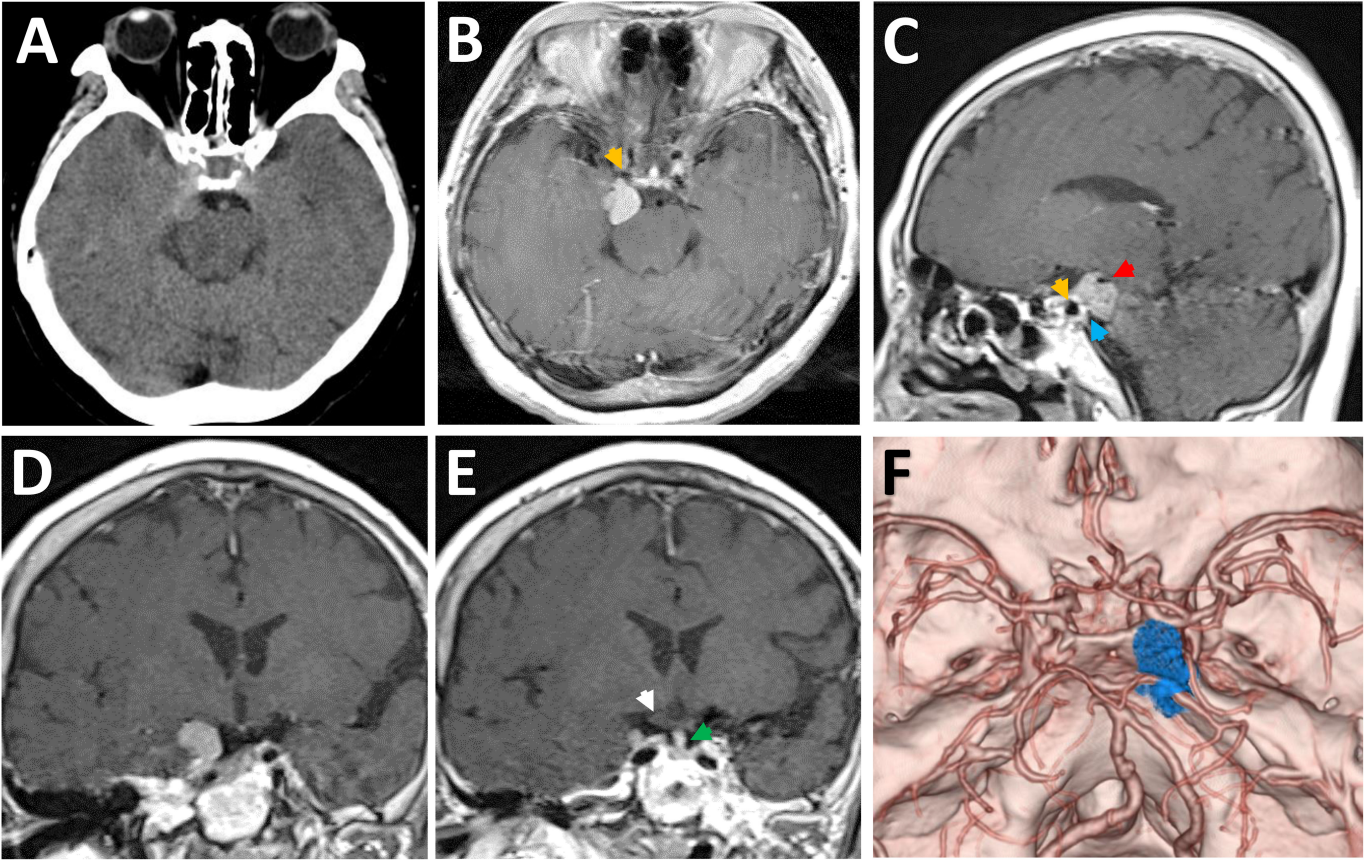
Radiological evaluation of the retrosellar lesion preoperatively. (A) Preoperative CT image showing a hyperdense lesion on the right-sided of posterior clinoid process. (B-E) Post-contrast (gadolinium-enhanced) axial (B), sagittal (C), and coronal (D, E) MRI images showing the thick enhancement of the lesion as well as the relative position to surrounding neural structures. (F) Preoperative CT angiography images demonstrating the relative location of tumor to the Willis’ circle and the dorsum sellae. The yellow arrows denote the posterior bend of caveronous carotid artery. The red arrow indicates the posterior cerebral artery. The white arrow denotes the up-elevated right optic chiasma. The green arrow indicates the pituitary stalk. The blue arrow indicates the dural detachment of the tumor on the posterior clinoid process.
